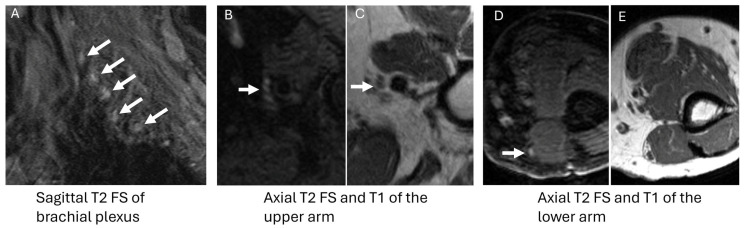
A 75-year-old male with a history of IgM monoclonal gammopathy presents with progressive numbness, paresthesias, and weakness in his hands. MRI showed diffusely increased T2 signal with multifocal areas of neural thickening in the brachial plexus ((A), arrows) and peripheral nerves of the upper arm ((B–E), white arrows). Left median nerve biopsy showed increased demyelination with inflammatory cells, suggesting an immune-mediated demyelinating neuropathic process.
